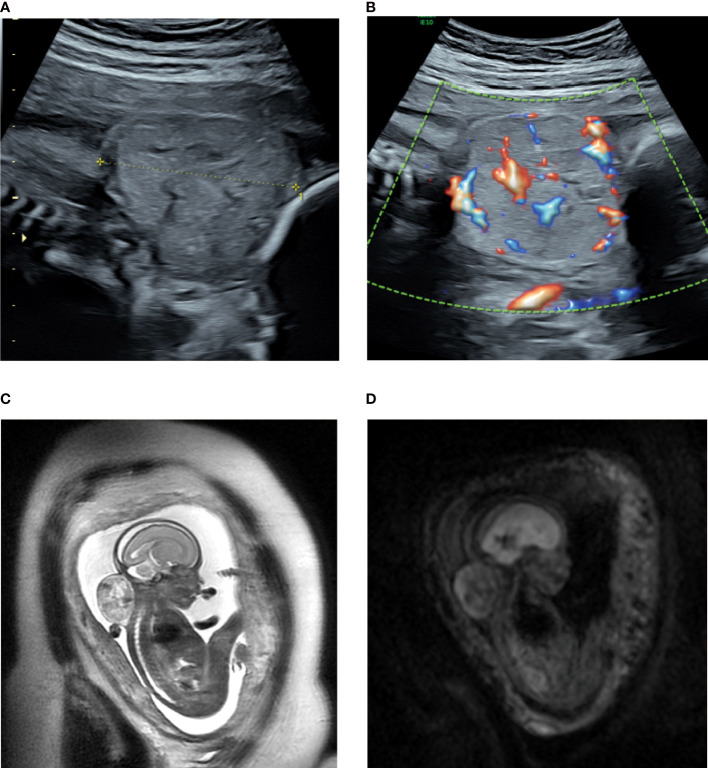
(A, B) Sonogram of the fetus with a posterior neck tumor at 23 weeks of gestation. (A) sagittal section; (B) color Doppler flow imaging; (C, D) Magnetic resonance multiple sequence imaging of the fetal neck tumor at 23 weeks of gestation. (C) sag T2SSFSE; (D): Ax DWI b = 600.
